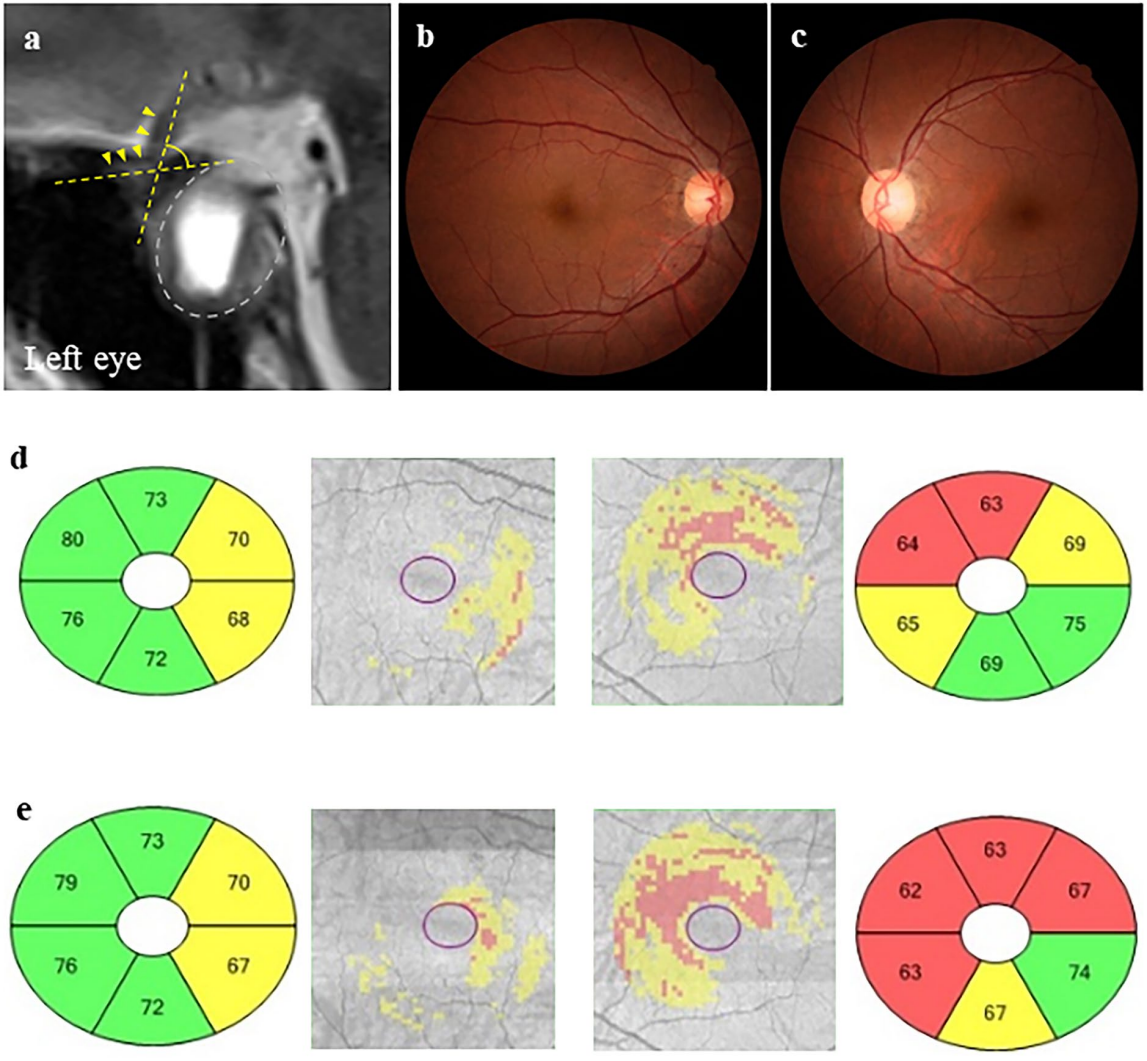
Representative clinical findings of a case with good visual outcome (case 1). A 63-year-old woman with pituitary adenoma with optic nerve bending in the left eye. Preoperative and postoperative best-corrected visual acuities (BCVAs) were − 0.08 and − 0.08 in the right eye and 0.82 and − 0.08 in the left eye logarithm of the minimum angle of resolution units, respectively. (a) Preoperative sagittal T2-weighted magnetic resonance images. The optic nerve–canal bending angle (ONCBA) was formed by the optic nerve (nerve indicated by yellow arrowheads) in the optic canal and the optic nerve in the intracranial subarachnoid space at the optic canal’s exit (angle indicated by yellow dotted lines). White dotted lines indicate a tumor. The ONCBAs of this case were 31° in the right eye and 76° in the left eye. Therefore, the left eye is the one with optic nerve bending. (b, c) Color fundus photograph showing the normal appearance at preoperative visit (B, right eye; C, left eye). (d, e) Ganglion cell layer (GCL) + inner plexiform layer (IPL) deviation map showing the expansion from the parafovea to the periphery of the red area indicates the decrease of GCL + IPL thickness and outside normal limits. GCL + IPL deviation map and sector graph of the right eye (left 2 panels) and left eye (right 2 panels) show a decrease in the macular GCL + IPL thickness, especially from nasal to superior sectors in the left eye. A slight decrease in postoperative GCL + IPL thickness is observed in the left eye (d, preoperative; e, postoperative visit).
